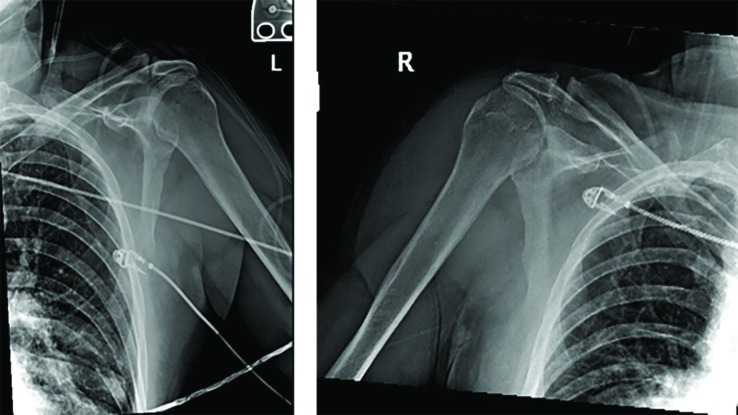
Radiography taken after second attempted reduction, which demonstrates successful reduction
